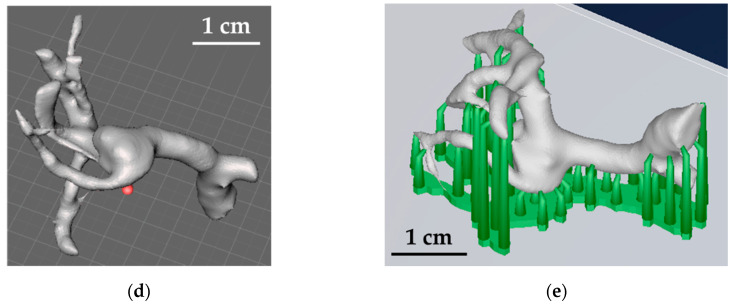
Elaboration of the STL file starting from the DICOM image of a cerebral aneurysm structure: (a) CTA image; (b) conversion to the first STL file; (c) first simplification of the model; (d) second simplification of the model in order to be limited to the surrounding of the aneurysm to speed up the printing process; (e) creation of the supports (green structures) and slicing.
